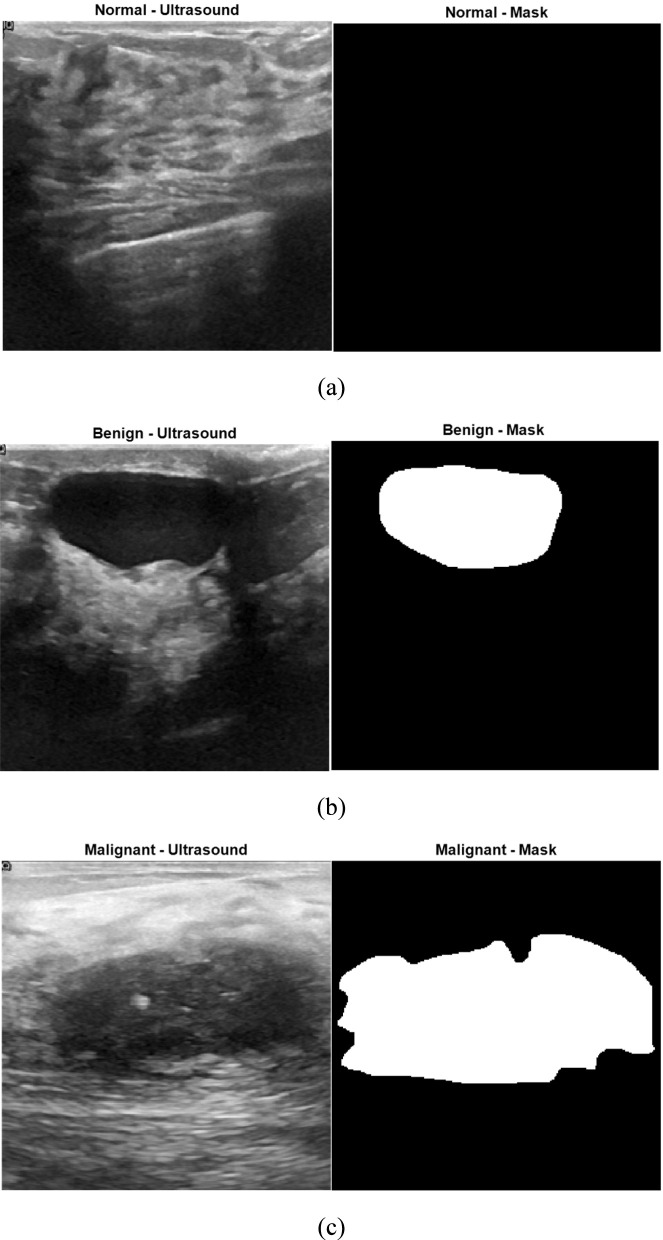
Example ultrasound images and corresponding binary masks for the three dataset classes: (a) normal, (b) benign, and (c) malignant (Al-Dhabyani et al., 2020).
